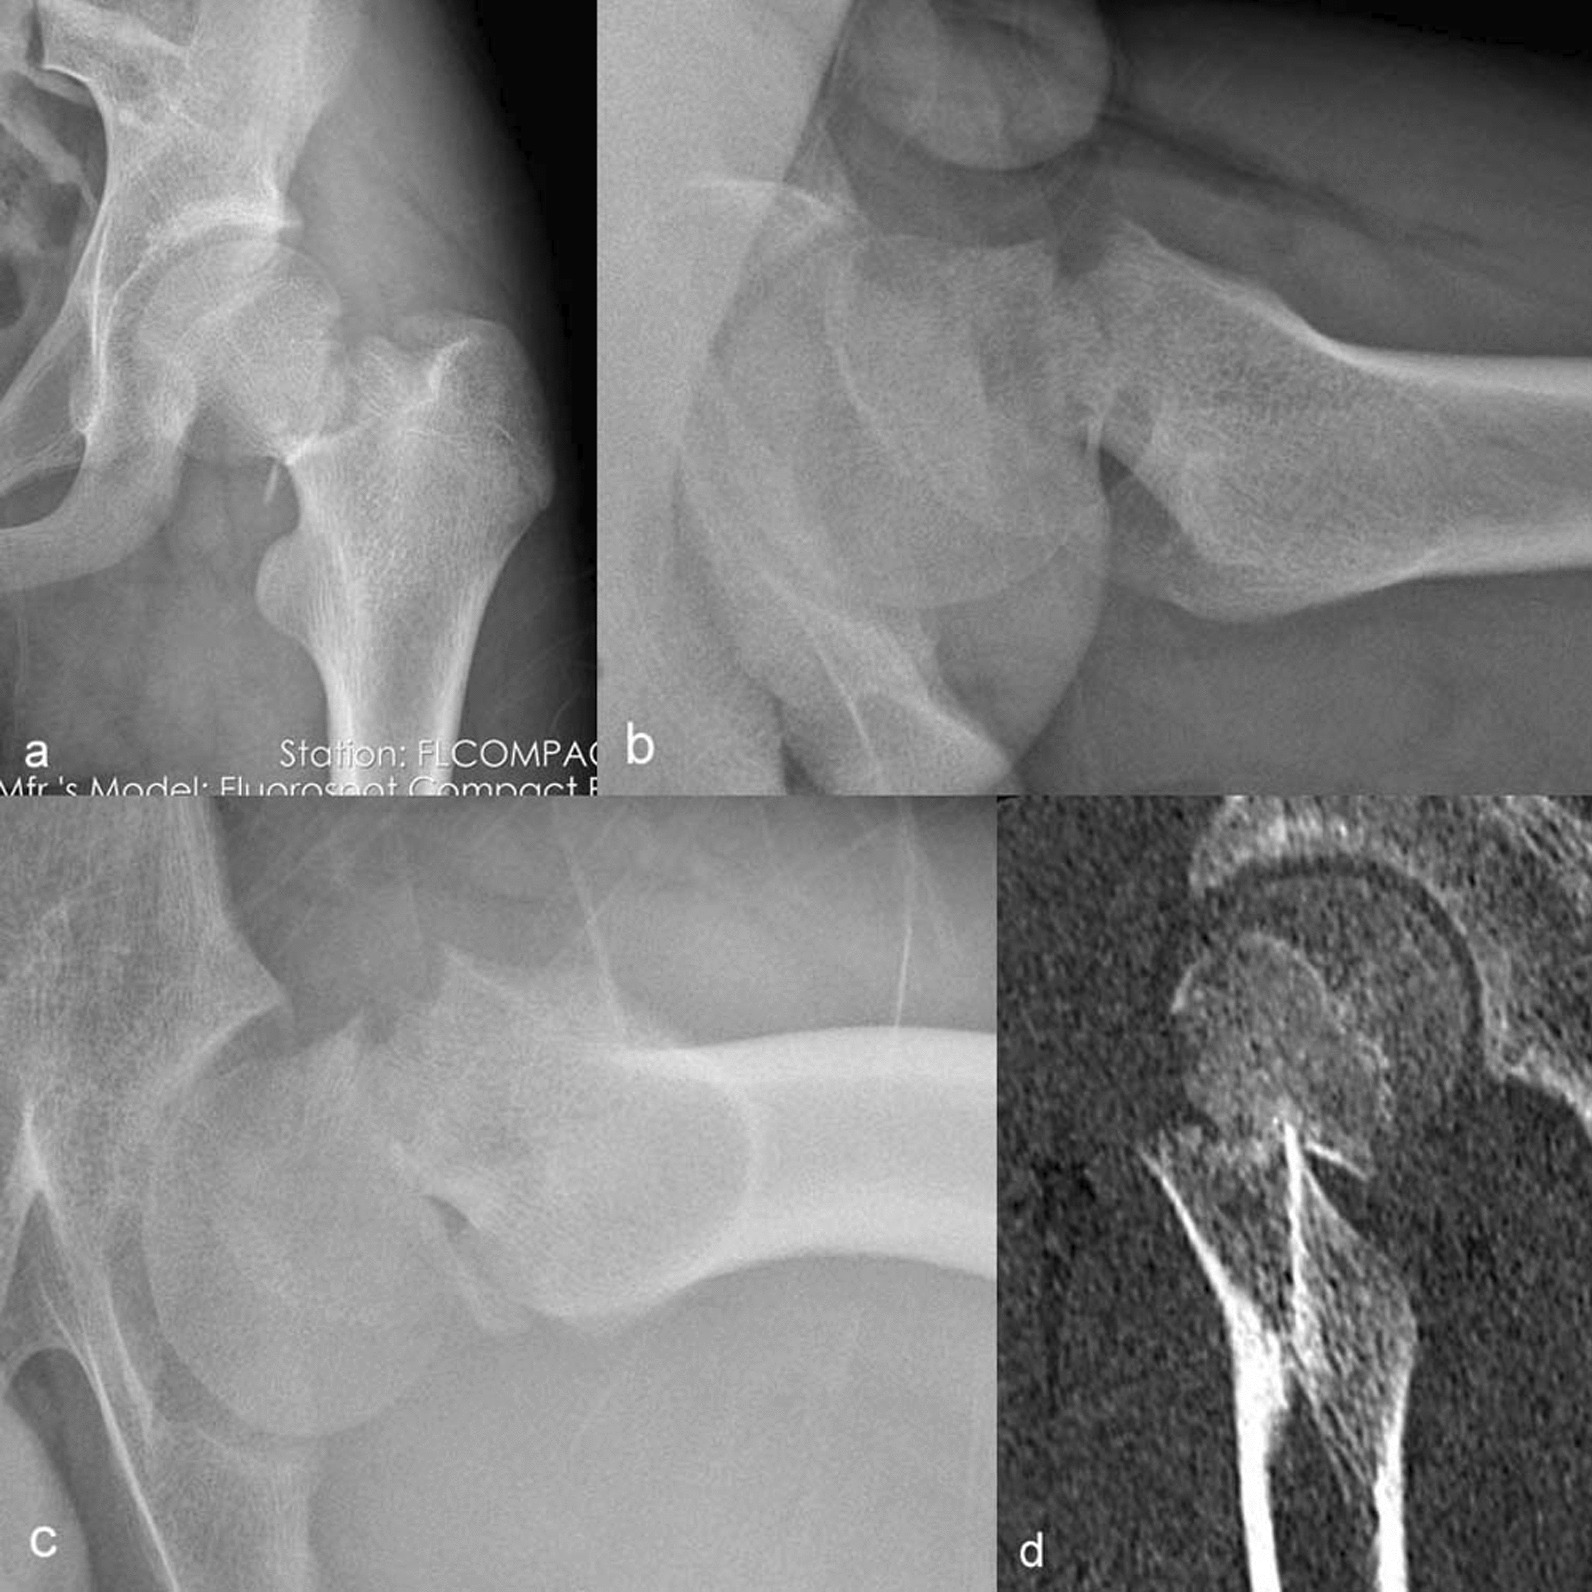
Case 2, a 18-year-old male, admitted to hospital one day after trauma. a AP view shows complete fracture of femoral neck. b Cross-table lateral view shows angular dislocation at the broken end. c Modified lateral view shows more obvious angular dislocation at the broken end than in the cross-table lateral view. d CT imaging confirms that the broken end is obviously misaligned and angulated
